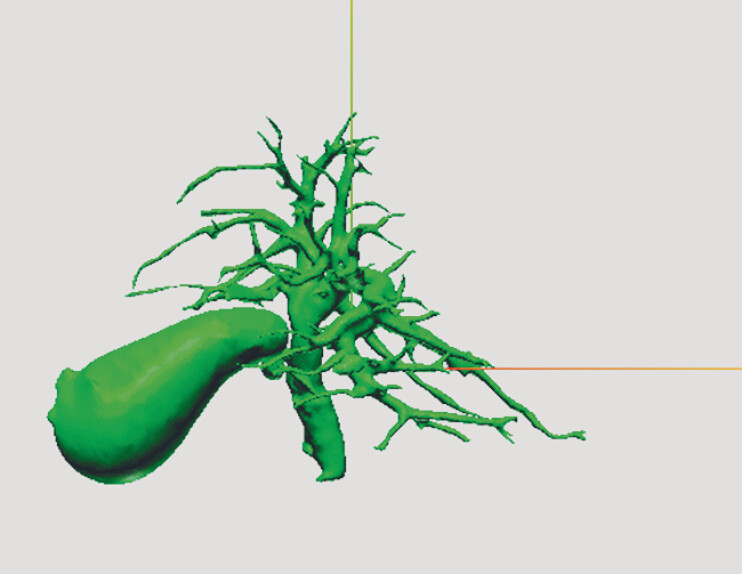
Magnetic resonance imaging was converted into a three-dimensional anatomical model.
